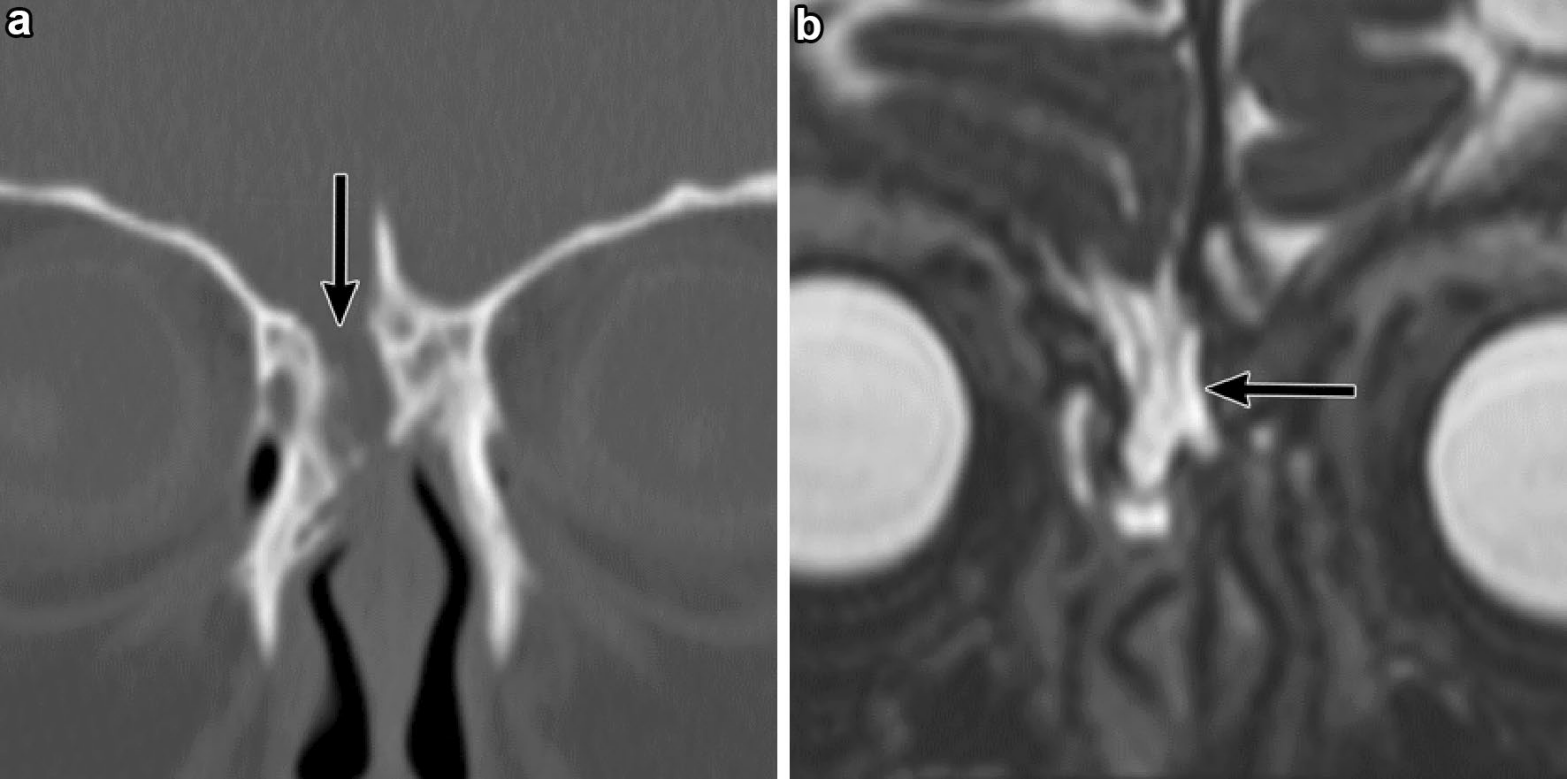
Multiple skull base fractures in a 3-year-old boy with recurrent bacterial meningitis. The patient had a head injury in a traffic accident 2 years prior to presentation. a Coronal computed tomography shows that the cerebrospinal fluid space communicates with the ethmoid antrum through a partial defect of the cribriform plate (black arrow). b Magnetic resonance (MR) cisternography (coronal T2-weighted MR image) shows the meningocele (black arrow) protruding through a defect of the cribriform plate. Although not shown here, there were also fractures in the left temporal bone
